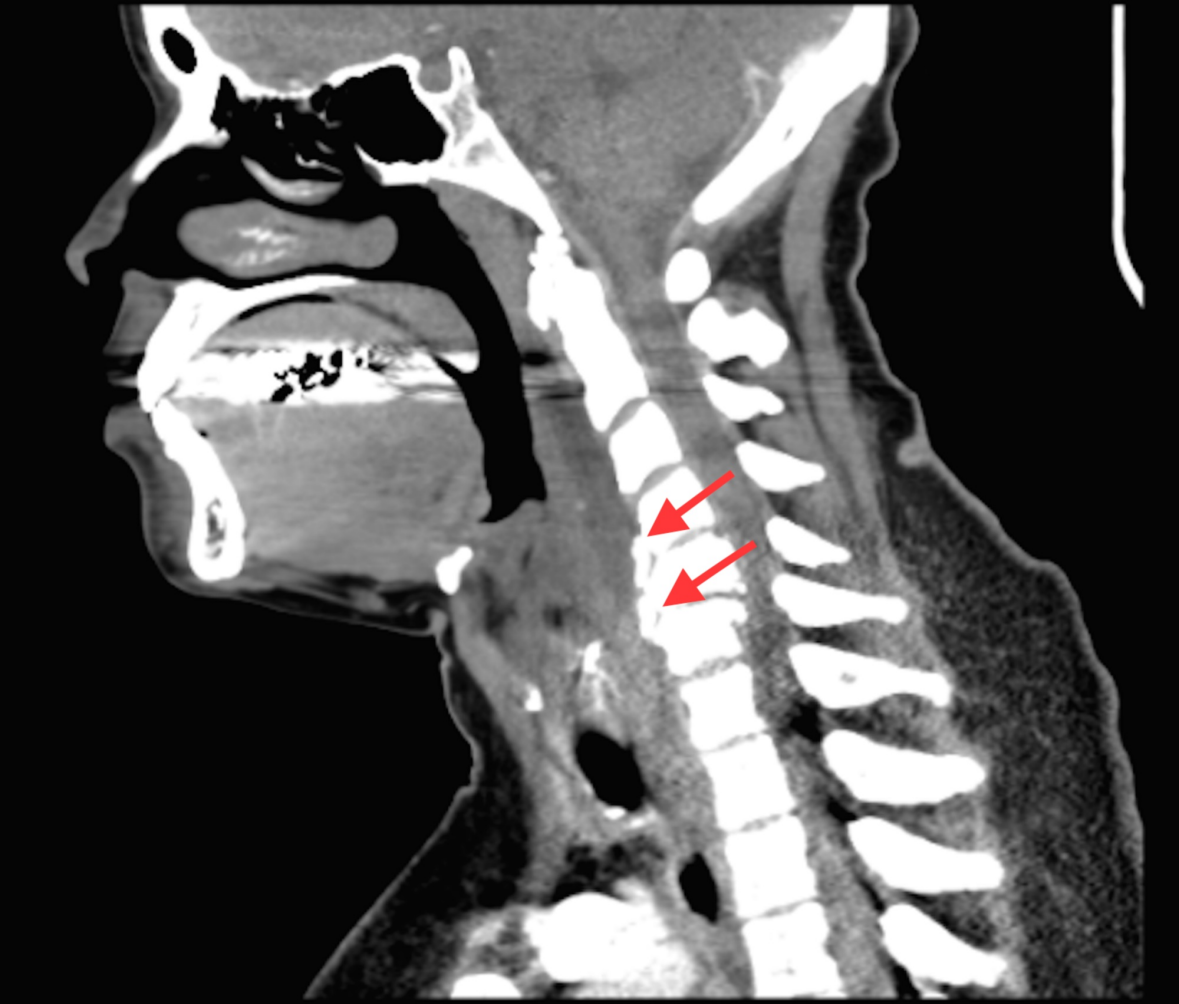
Sagittal view of CT cervical spine showing degenerative changes at C4-C5, C5-C6, calcific tendonitis of the longus colli (arrows)
